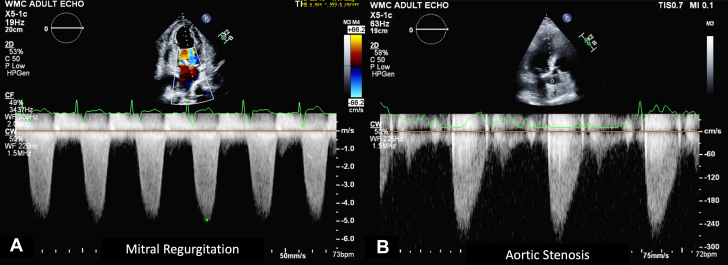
Comparing Mitral Regurgitation and Aortic Stenosis Doppler Profiles (A) Mitral regurgitation Doppler showing rounded jet contour that occurs during both the isovolumic contraction time and isovolemic relaxation time periods of the cardiac cycle. (B) Compared with aortic stenosis, Doppler that shows a rounded but slightly peaked velocity that occurs in systole after isovolemic contraction time but before isovolemic relaxation time.2.Be sure to rule out other etiologies for outflow obstruction including both subvalvular and supravalvular stenosis. A subaortic membrane is a thin membrane that may be discrete or tunneled in the LVOT. When zooming in on the LVOT, look for a membrane and check for flow acceleration with color Doppler that occurs in the LVOT (below the AV). It is not uncommon to see associated aortic regurgitation in these patients. In patients with subvalvular narrowing from hypertrophic cardiomyopathy, the thickened ventricular septum narrows the LVOT. Systolic anterior motion of the anterior mitral valve leaflet may also be present leading to LVOT turbulence by color Doppler in the location of the narrowing and septal annular motion. The CW Doppler has a late peaking dagger-shaped appearance (Figure 17). PW cursor placement is extremely important in these patients with careful adjustment to avoid flow acceleration (Doppler aliasing) from the subvalvular obstruction.
